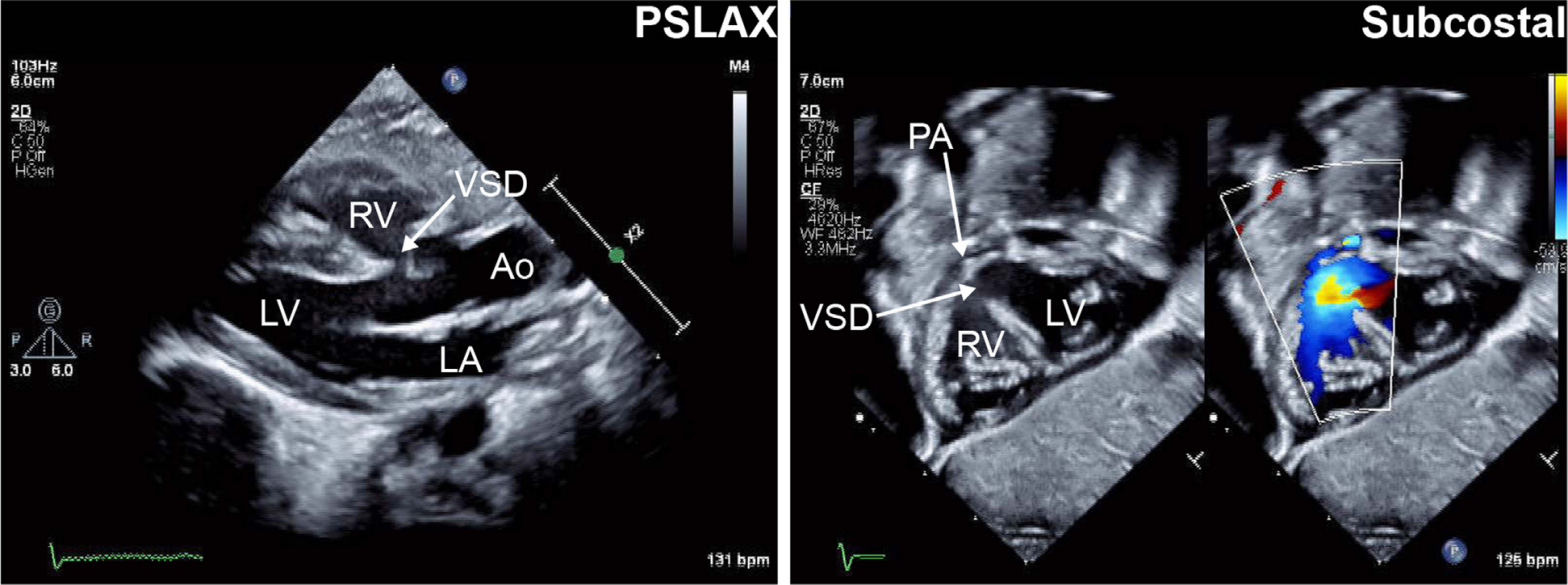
Echocardiograms Demonstrating Tetralogy of Fallot With Severe Pulmonary Stenosis Parasternal long axis and subcostal sagittal images demonstrate the patient’s anatomy. Color flow imaging in the subcostal image shows severe subpulmonary and pulmonary stenosis and right to LV level shunt. Depth (cm) is indicated to the right of each image. Ao = ascending aorta; LA = left atrium; LV = left ventricle; PA = pulmonary artery; RA = right atrium; RV = right ventricle; VSD = ventricular septal defect.
